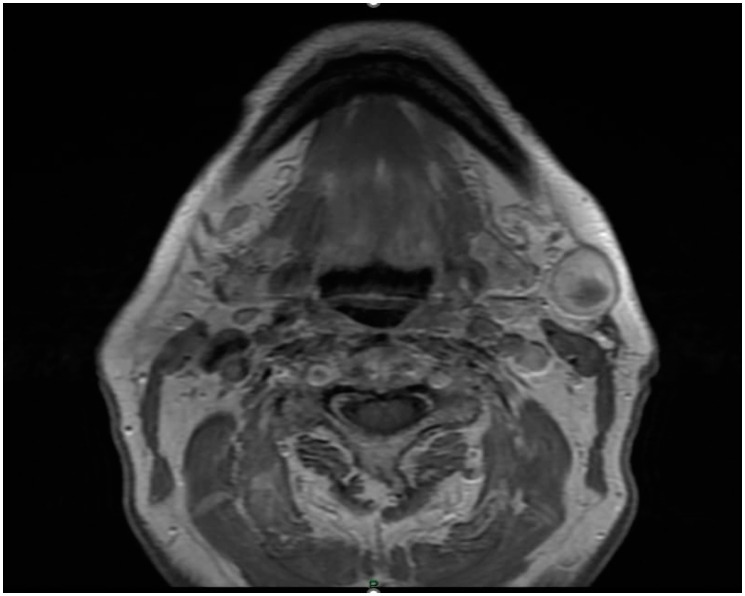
MRI image: a nodulation of approximately 2.3 × 1.6 cm is observed, solid with an internal central area showing fluid signal.
